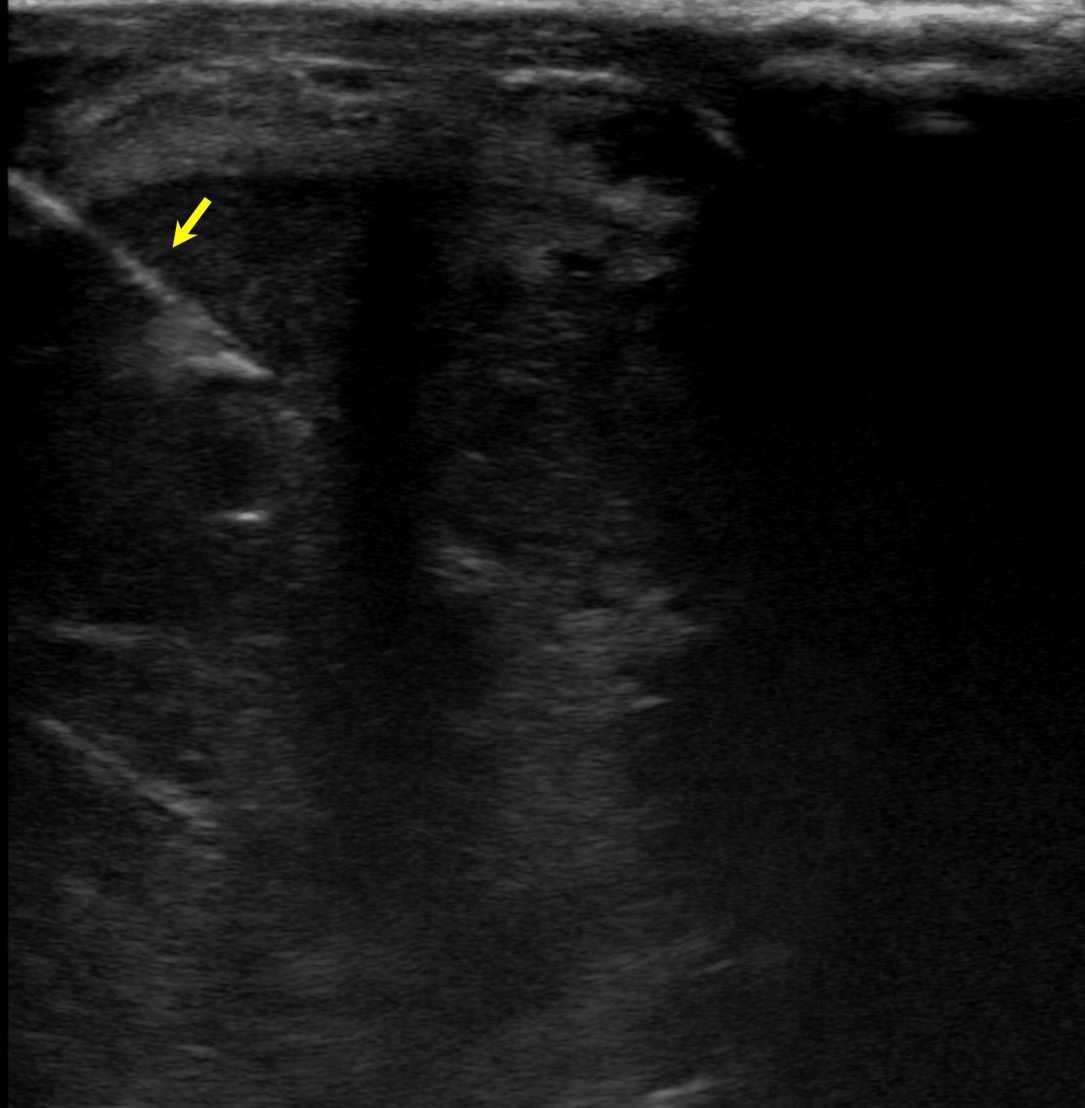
Ultrasound demonstrating 18-gauge BioPince needle (arrow) positioned in the penile lesion for core biopsy
